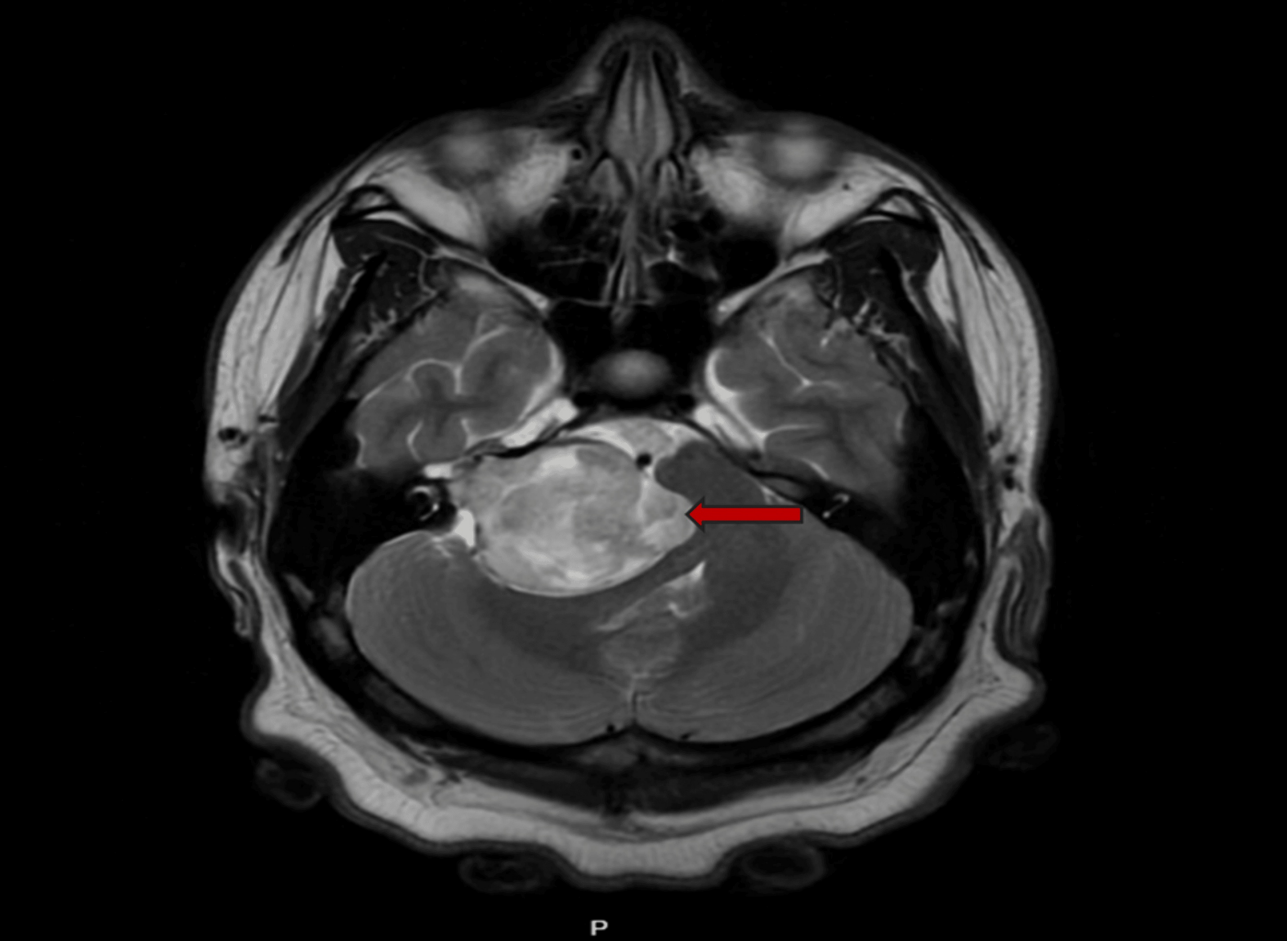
Magnetic Resonance Imaging (MRI) of the Internal Auditory Canal MRI of the internal auditory canal shows a homogenously high T2/FLAIR signal intensity mass centered within the right lateral cerebellopontine angle with direct extension into the right internal auditory canal. The mass measures approximately 4.9 x 4.2 x 4.2 cm (red arrow). There are mass effects and effacement of the lateral aspect of the pons and midbrain, along with near-complete effacement of the 4th ventricle with hydrocephalus. FLAIR: Fluid-Attenuated Inversion Recovery
